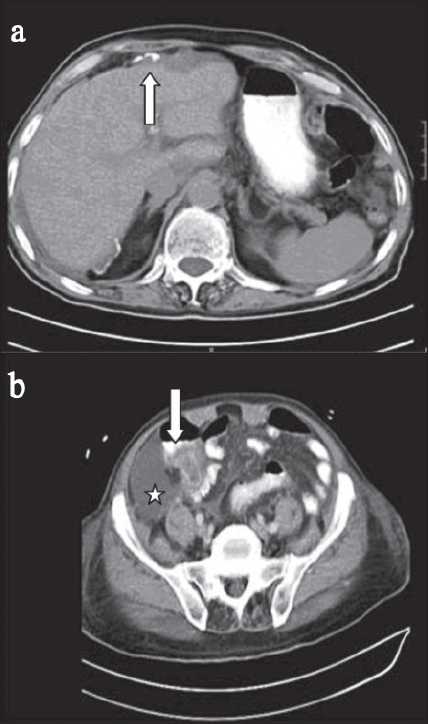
Axial CT scan of the abdomen and pelvis with oral and IV contrast (a) Intraperitoneal fluid collection seen at the right subdiaphragmatic space with calcifications (arrows). (b) Heterogeneous mass at the cecum and appendix (open arrow) with free fluid collection (*)
